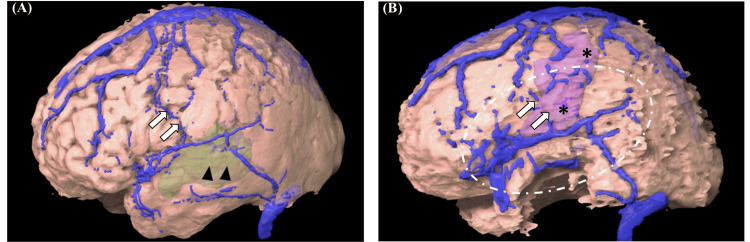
Three-dimensional images of the brain Three-dimensional images created from pre- (A) and intraoperative (B) MRI data are presented. Arrowheads and asterisks indicate enhanced tumors (translucent) and hemorrhagic infarctions (translucent), respectively. The central veins that drain the precentral gyrus near the Sylvian fissure (arrows) were difficult to visualize under compression (dotted circle area) on intraoperative MRI (B). MRI: magnetic resonance imaging
